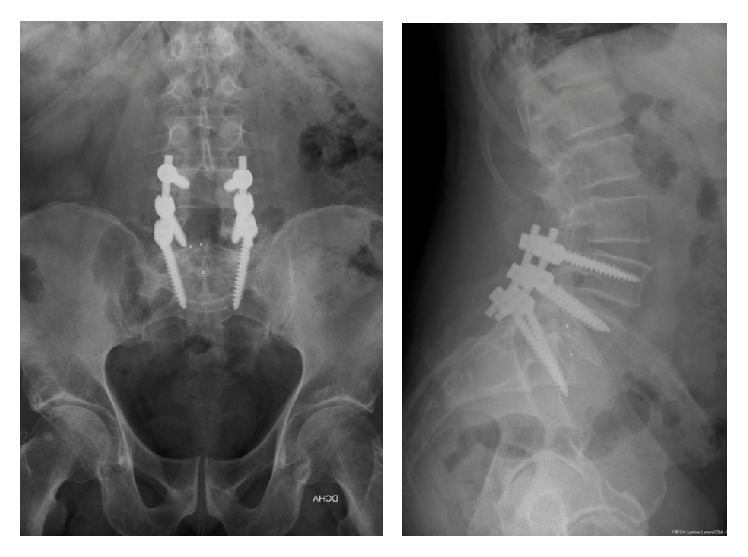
Postoperative X-ray. One-year follow-up.
